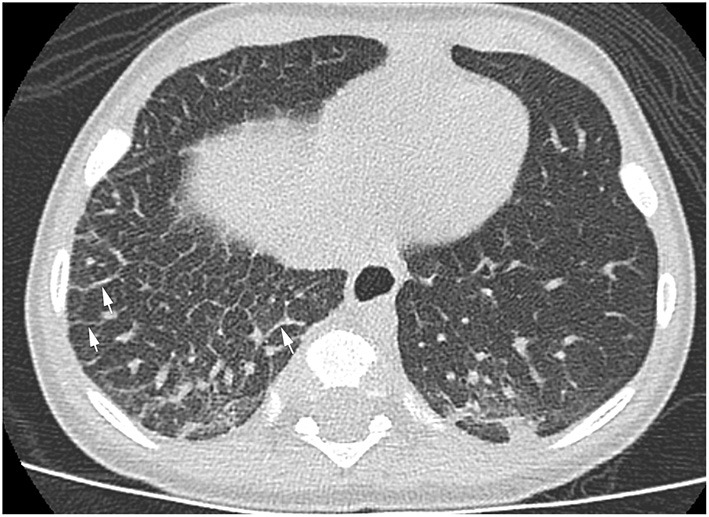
High resolution chest CT showing diffuse thickening of the interlobular septae (white arrows) in the right lung.
